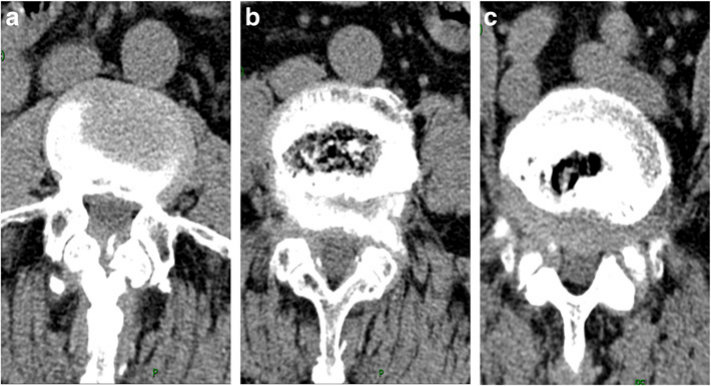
a, b, c Plain computed tomography image of the lumbar intervertebral discs shows L3/4, L4/5, L5/S1 disc herniations, spinal volume reduction, and nerve compression
